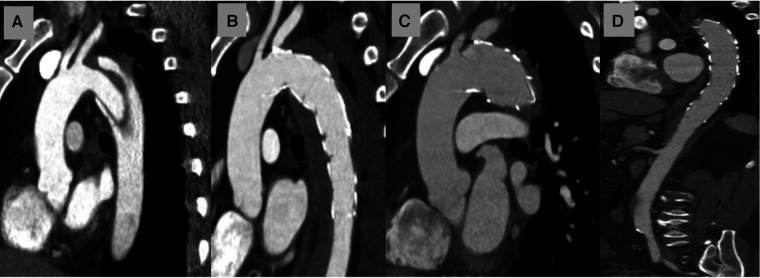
(A) Shows the preoperative sagittal CT scan at the time of the type B dissection diagnosis in a patient with intramural hematoma (at the site of the left subclavian artery). (B) demonstrates the postoperative sagittal CT scan after TEVAR in the landing zone II. (C) indicates the sagittal CT scan of the same patient: the stability of the proximal endograft position is well visible. The remodeling of the downstream aorta is shown in (D).
